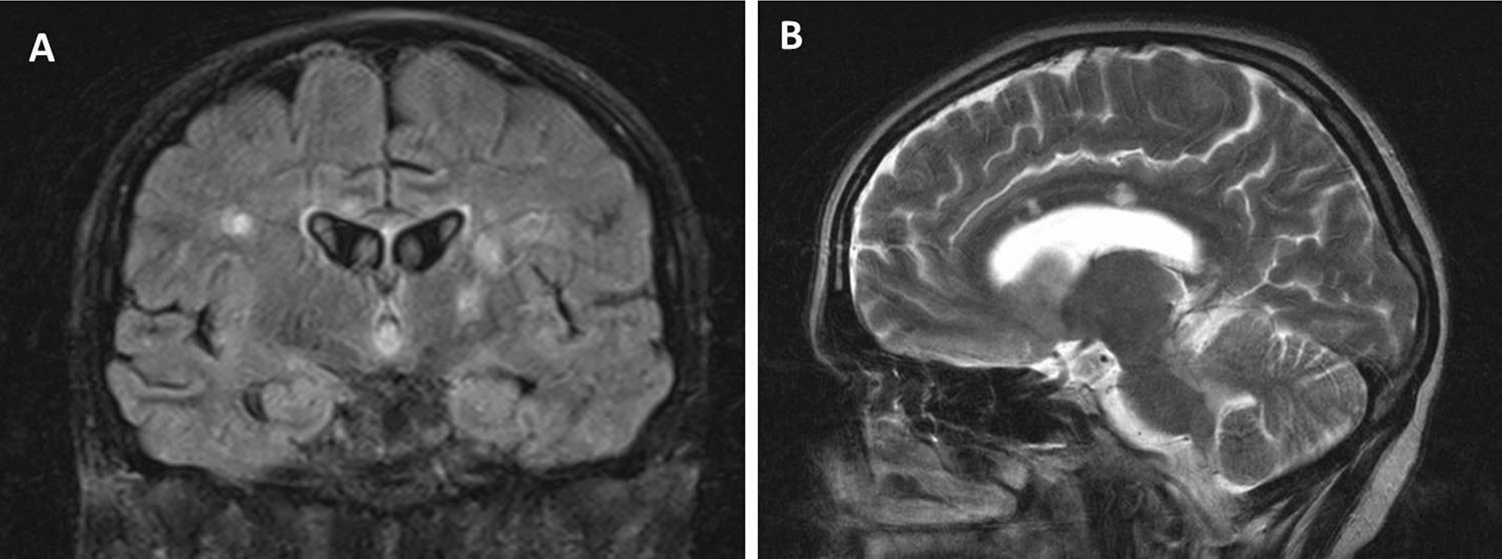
MRI changes in Susac syndrome MRI sequences of showing callosal (arrows), periventricular and thalamic lesions in coronal FLAIR (A) and sagittal T2W (B) images
